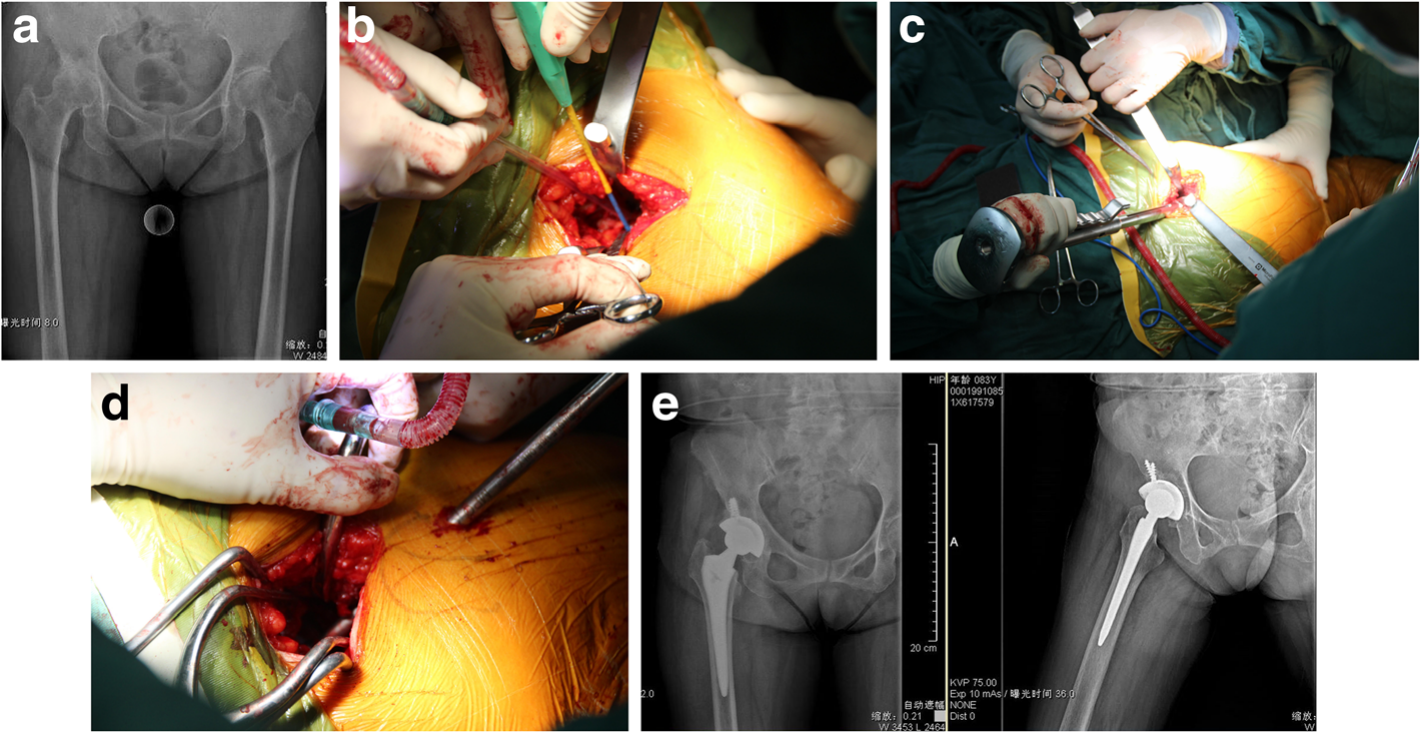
A patient suffered from right hip osteoarthritis (a). Following the initial incision, two wing-tipped elevators were used to split the gluteus maximus muscle and expose the underlying gluteus medius muscle (b). Sequential femoral broaches were then used to complete preparation and size the proximal femoral canal (c); use an appropriately sized acetabular basket reamer to ream the acetabulum through the main incision and connected to the reamer drive shaft inserted through the cannula proximally into the main incision through a 1-cm incision located 1 to 2 cm posterior to the femur (d). Postoperative standard anteroposterior and lateral radiographs (e)
